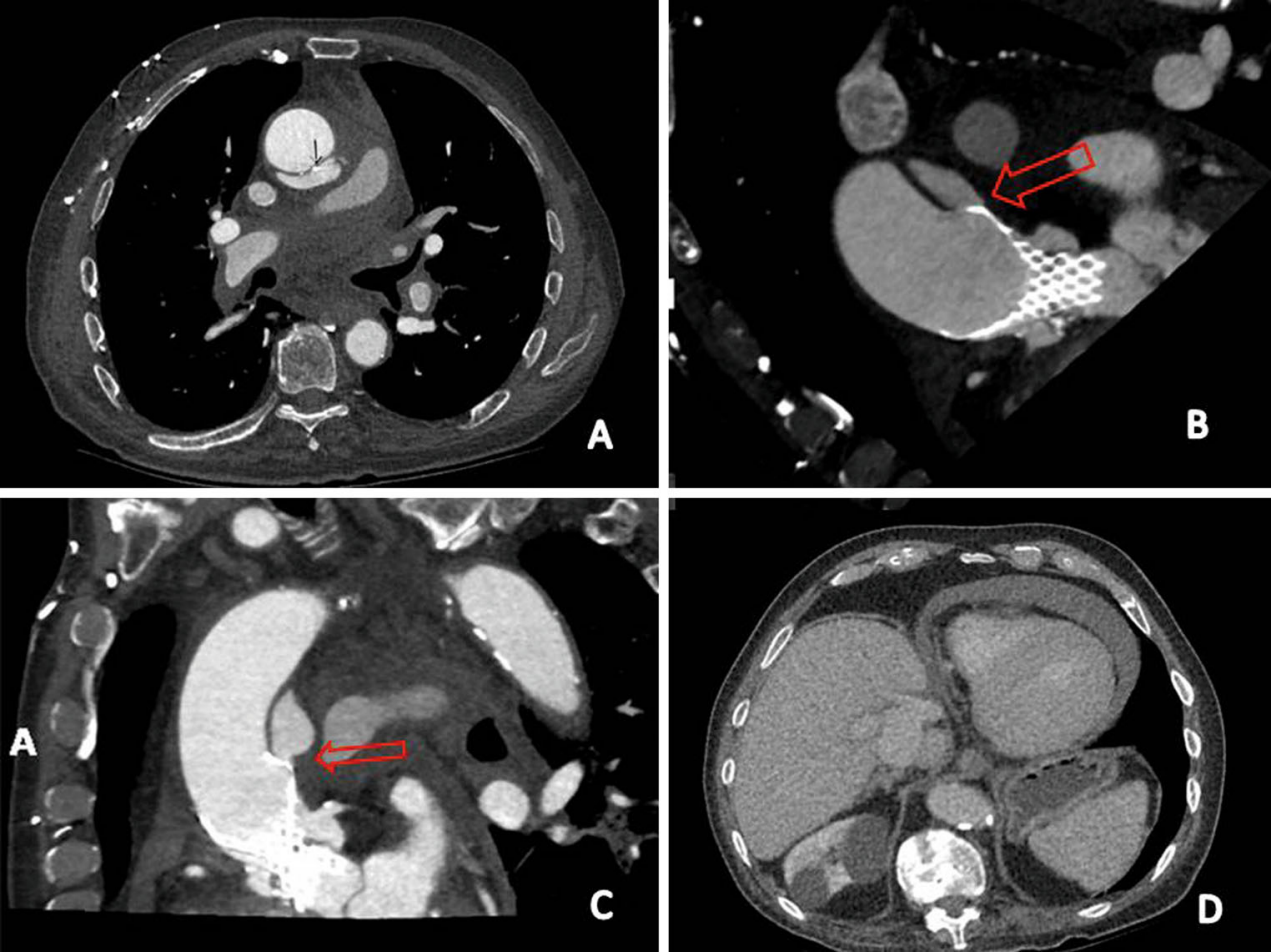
a periaortic haematoma and contrast extravasation at the level of the distal site of the prosthesis. b and c The distal stent strut was located outside the ascending aorta towards the pulmonary artery continuation. d CT confirmed the echocardiographic view of pericardial effusion
